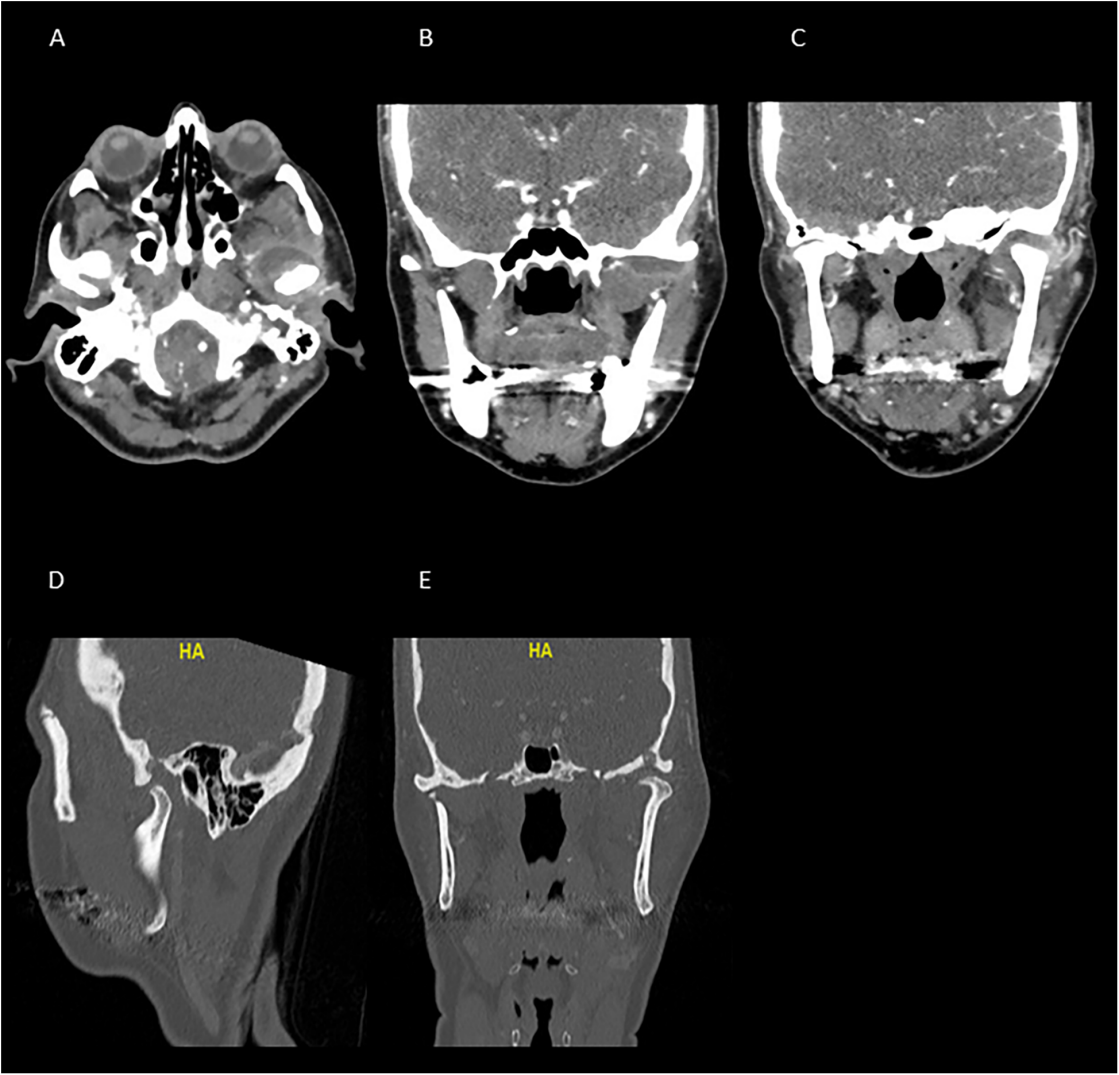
CT scan taken upon arrival to the emergency department. The images (A–C) show a significant hypodense area at the anterior part of the left TMJ, demonstrating signs of inflammation, edema, and collection around the left joint. (A) Axial view, (B,C) coronal view. The images (D,E), demonstrate an erosion of the glenoid fossa and the significant bone involvement, suggesting the presence of an advanced pathological condition. Images (D,E) show the sagittal and coronal views, respectively, at the bone window.
